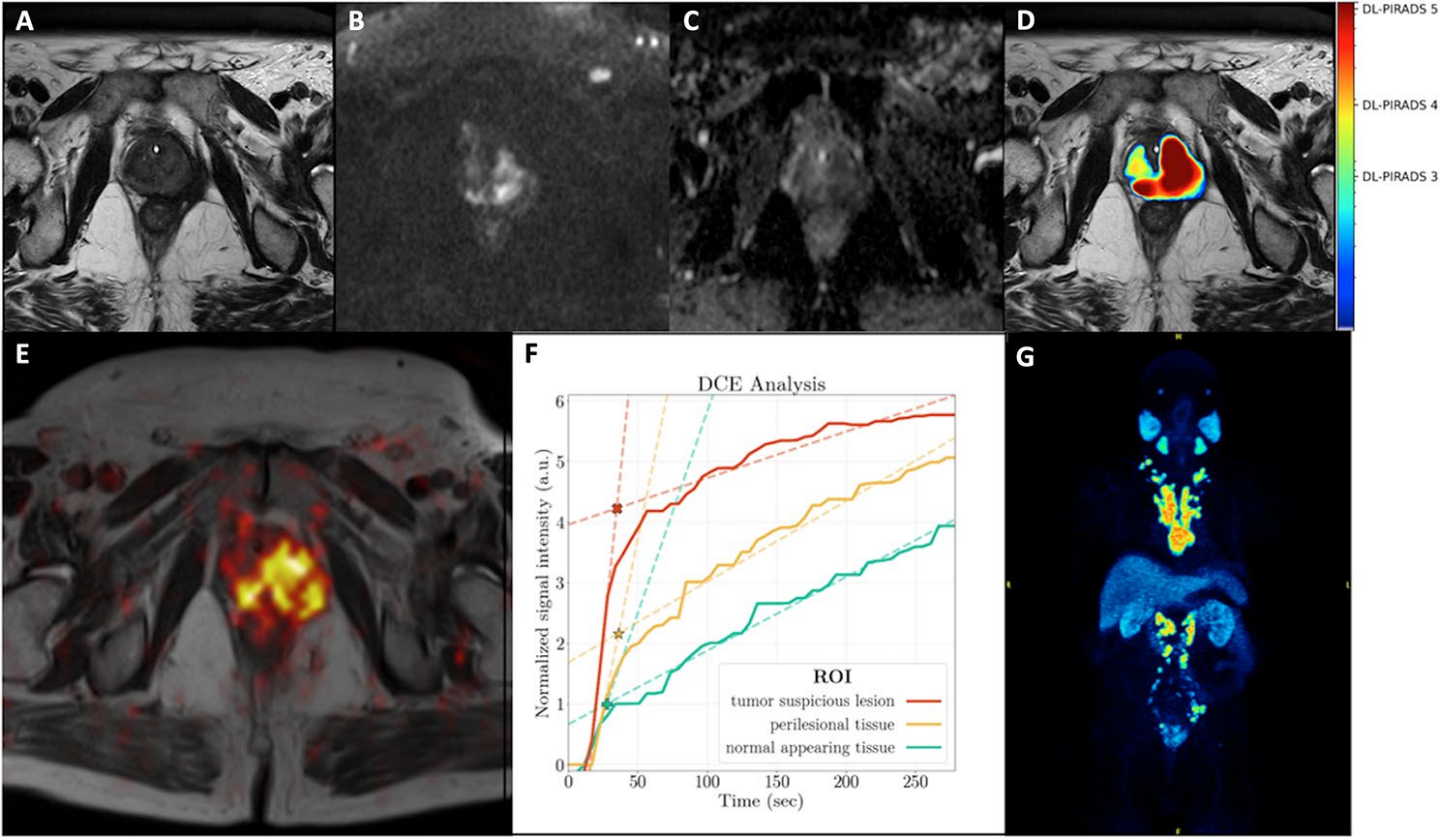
A 77-year-old patient with an initial diagnosis of PC (Gleason score 9) referred for initial staging with PET-MRI. His PSA level at the time of examination was 18.9 ng/ml. Distinct T2w hypointensity of the prostate (A) with marked diffusion restriction with signal increase in the high b-values (B) and low ADC values in the ADC-map (C) predominantly in the left hemisphere of the prostate with extension over the midline to the right side and with extracapsular extension compatible with a PIRADS score of 5. The AI-based PI-RADS equivalent (DL-PIRADS) is in good agreement with the MRI findings and also shows a very high probability score for PCa (DL-PIRADS 5, D). Correspondingly, [^18^F]-PSMA-1007 PET (fused T2w, (E)) shows an intense [^18^F]-PSMA-1007 accumulation in the prostate gland. Quantitative DCE data based on manual segmentation showed a steeper wash-in slope for tumor-suspicious lesion (TSL, red) compared to perilesional tissue (PLT, yellow) and normal appearing tissue (NAT, green). The corresponding fitted maximum (*, intersection of wash-in and wash-out slope) was 4.2 for TSL, 2.2 for PLT and 1.0 for NAT, allowing a clear discrimination between them. All 3 curves showed an increasing curve pattern (F). Whole-body [^18^F]-PSMA-1007 PET imaging showed the primary tumor, multiple iliac, retroperitoneal, mediastinal and cervical lymph node metastases, as well as bone metastases in the sacral bone and the thoracic spine (G)
